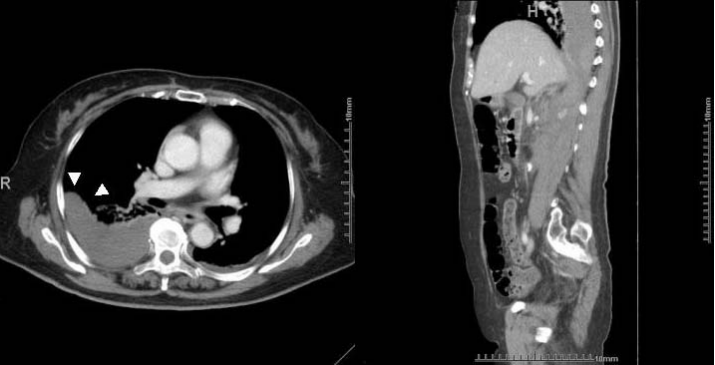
(a) Thoracic computed tomography performed 3 h after admission (axial image:left); this CT scan shows a right extrapleural hematoma. One part of the thoracic hematoma shows a D-shaped opacity (Δ). (b) Sagittal-reconstruction computed tomography scan (right); This CT scan shows an enlarged 　　　　　　　　　　　　hematoma, extending from the right retroperitoneal space to the right extrapleural
